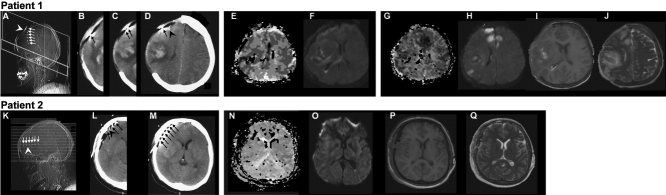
X-ray topographic images and cranial computed tomography (CT) slices of Patients 1 and 2 with individual locations of the electrocorticography (ECoG) strip electrodes (*arrows*, CT artifacts of electrodes) and the oxygen partial pressure probes (*arrowheads*) adjacent to strip electrodes. Please note that levels of axial sections differ somewhat among scans. The upper level shows Patient 1, with subarachnoid hemorrhage predominantly in the sylvian fissure with additional intraparenchymal hemorrhage and perifocal space-occupying edema. (A-D) A CT scan was performed after decompressive craniectomy with ECoG and tissue oxygen pressure (p_ti_O_2_) probe implantation 2 days after subarachnoid hemorrhage (SAH). (A) In the topogram, the arrows represent 6 single electrodes of the ECoG strip electrode. The arrowhead demarcates the p_ti_O_2_ probe. (B) The arrow indicates electrode 2. (C) Arrows indicate electrodes 3 and 4, respectively. (D) The arrow indicates electrode 5, and the arrowhead demarcates the p_ti_O_2_ probe. (E) Perfusion-weighted (PW) magnetic resonance imaging (MRI) and (F) diffusion-weighted (DW) MRI show no perfusion deficit or signs of cortical ischemia 4 days after SAH. The area of intracranial hemorrhage displays disturbance of the diffusion signal. (G) PW MRI and (H) DW MRI demonstrate areas of hypoperfusion and signs of cortical ischemia predominantly of the right frontal lobe, but also of the left frontal lobe 9 days after SAH. (I) MRI (T1 weighted) shows infarcted cortical tissue. (J) A T2-weighted MRI cross-section (more rostral) gives a detailed picture of the distribution of cortical infarcts with right frontoparietal and bifrontal representation. The lower level shows Patient 2, who suffered a subarachnoid hemorrhage from a ruptured aneurysm, which was clipped surgically 2 days after SAH. Cranial CT scan was performed 1 day after the craniotomy and ECoG and p_ti_O_2_ probe implantation. (K) X-ray topogram and (K-M) 2 different slices are depicted. (K) Six arrows indicate the ECoG strip with 6 single electrodes. The arrowhead demarcates the p_ti_O_2_ probe. (L) The arrows indicate electrodes 1, 2, and 3; the arrowhead indicates the p_ti_O_2_ probe between electrodes 2 and 3. (M) All 6 electrodes are displayed; however, the p_ti_O_2_ probe is not visible on this cross section. (N) Seven days after SAH, PW MRI showed no hypoperfusion, and (O) DW MR imaging revealed no signs of ischemia (disturbance of diffusion). (P) The same was true for the T1-weighted MRI and also (Q) for T2-weighted MRI (see Supplementary Clinical Results online for a more detailed description of the 2 individual clinical courses).
